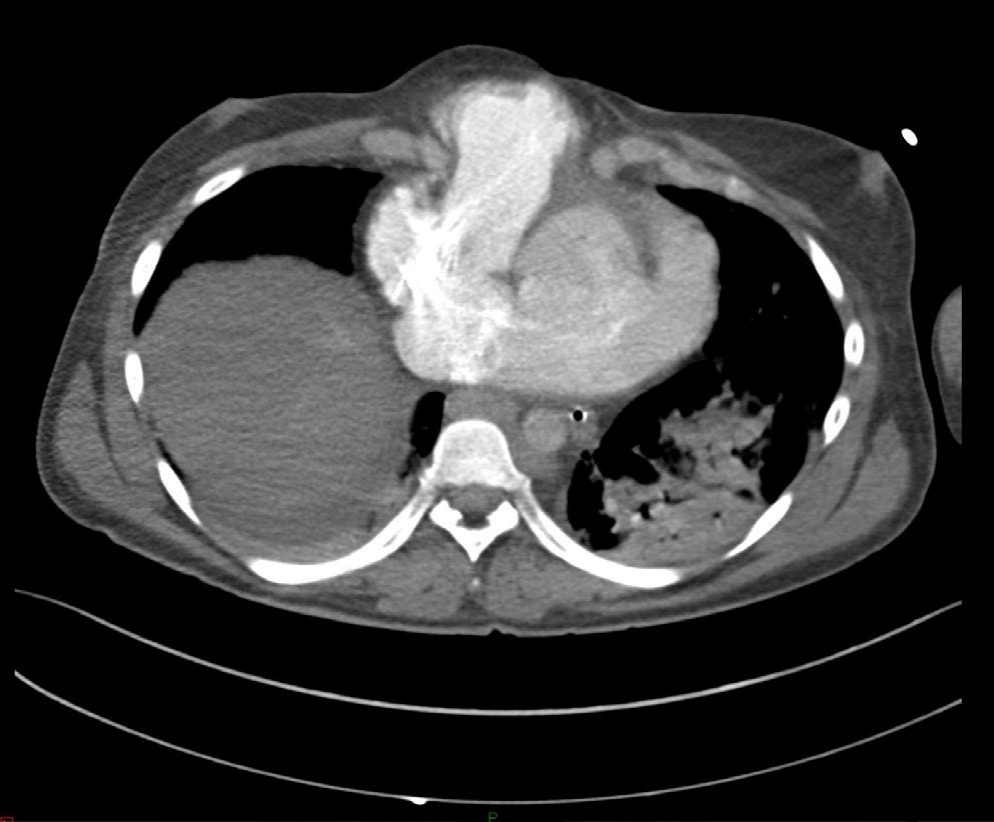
An enhanced computed tomography scan of the chest was performed to evaluate shortness of breath and chest pain. This shows partial ectopia cordis with right ventricular outflow tract herniation. The heart is seen extending through a midline defect in the sternum and lying outside the thorax, covered only by the skin. Atrial septal defect is also noted
